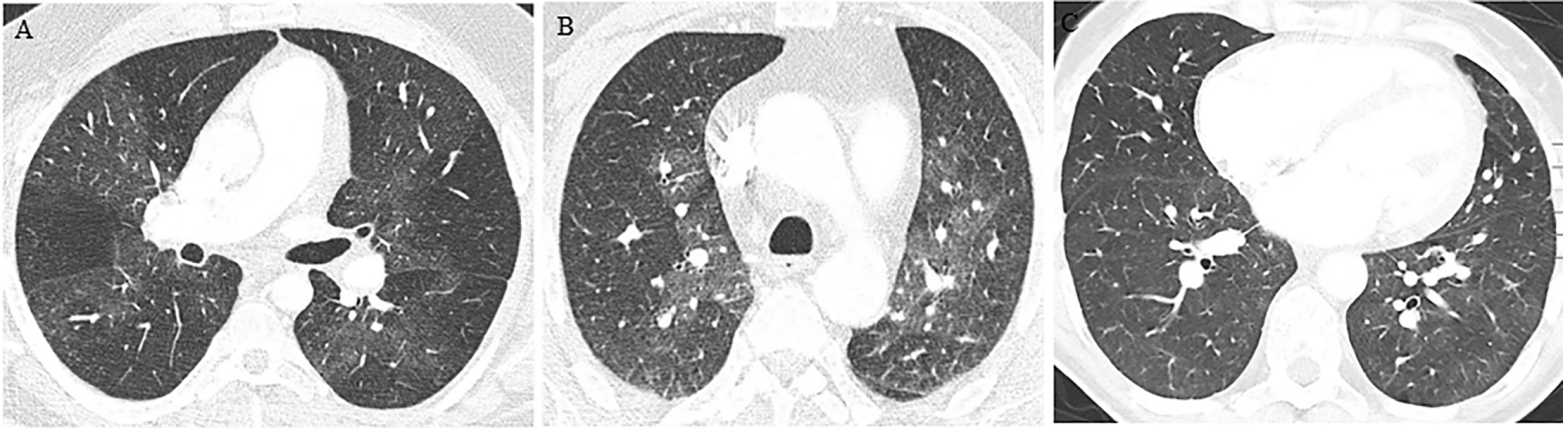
Mosaic perfusion in patients with CTEPH. (A) Axial CT image shows sharply demarcated segmental and subsegmental areas of hypo- and hyperattenuation (pattern 1). (B) Axial CT image shows perihilar areas of increased attenuation and vascularity with peripheral perfusion defects (pattern 2). (C) Axial CT image shows patchy heterogeneous lung attenuation (pattern 3).
